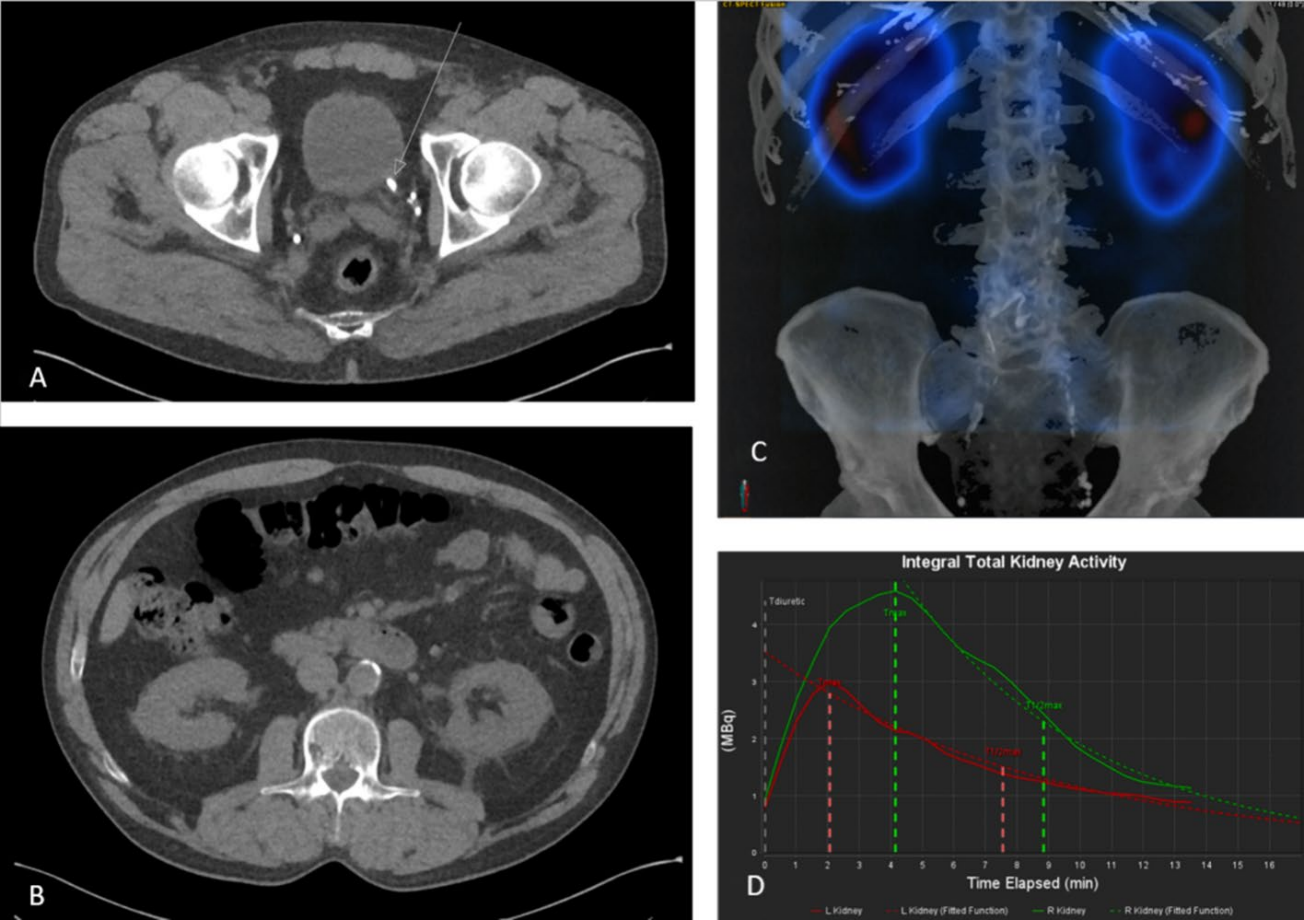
Images from case 11 in Table 1. The patient presented to the Emergency Department with renal colic symptoms, left flank pain and haematuria. A Axial UECT shows a stone in the left ureter close to the ostium with a diameter of 9 × 5 mm (arrow). B Axial UECT at kidney level shows hydroureter, fat stranding and thickening of the renal fascia in the left kidney. C 3D SPECT/CT reconstruction showing both kidneys with similar activity. D Bilateral falling renogram curves, red curve left kidney and green curve right kidney
